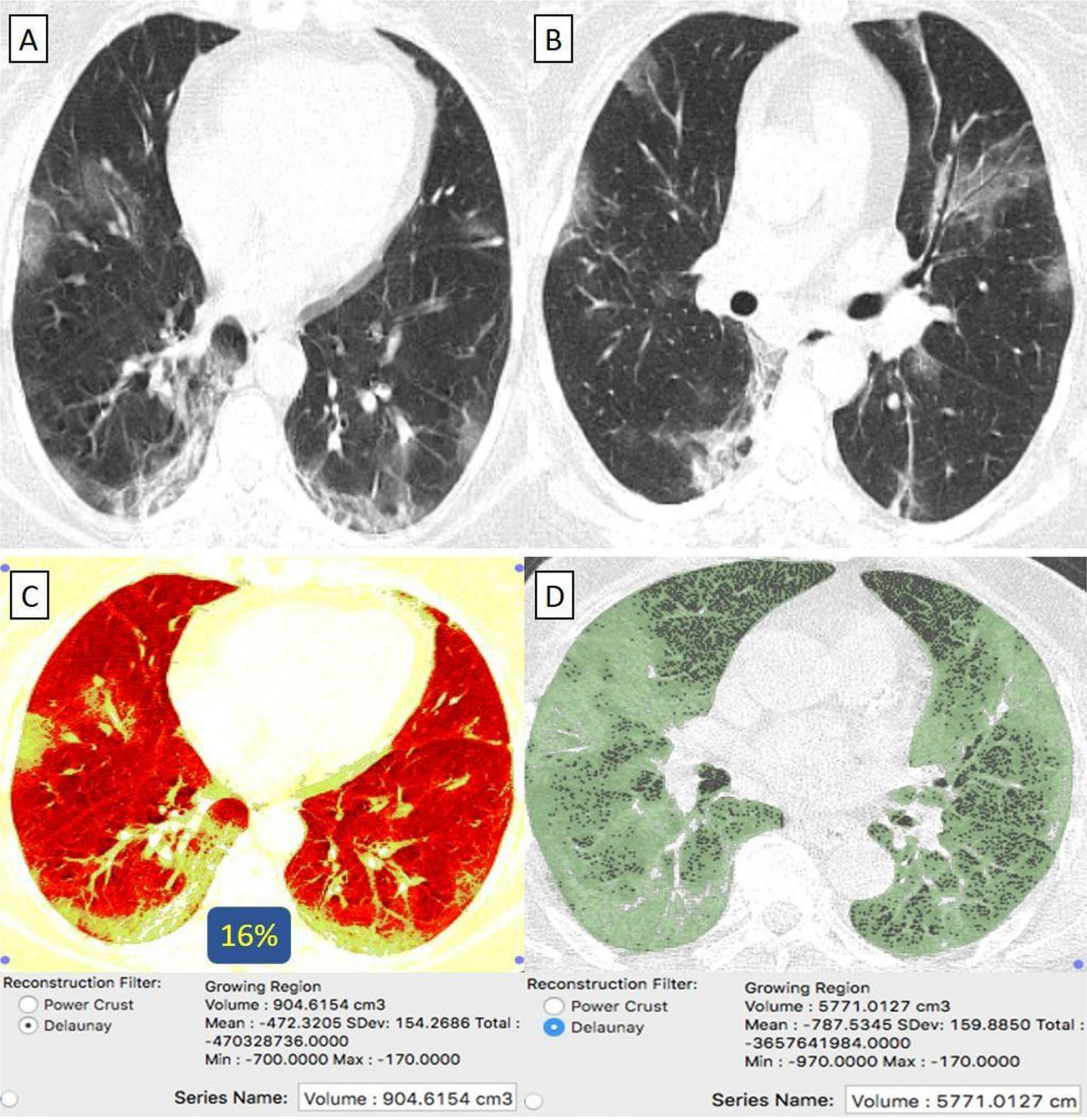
A 43-year-old female patient with negative past medical history and had received “Oxford-AstraZeneca” vaccine then got COVID-19 infection. A, B Axial chest CT cuts (lung window) showed bilateral mainly peripheral located ground-glass pneumonic patches and fine atelectatic bands. C CT-Volumetry of the pathological lung parts averages 904 cc. D CT-Volumetry of total lung volume averages 5771 cc. So, only 16% of the lung parenchyma was diseased (Score 1)
